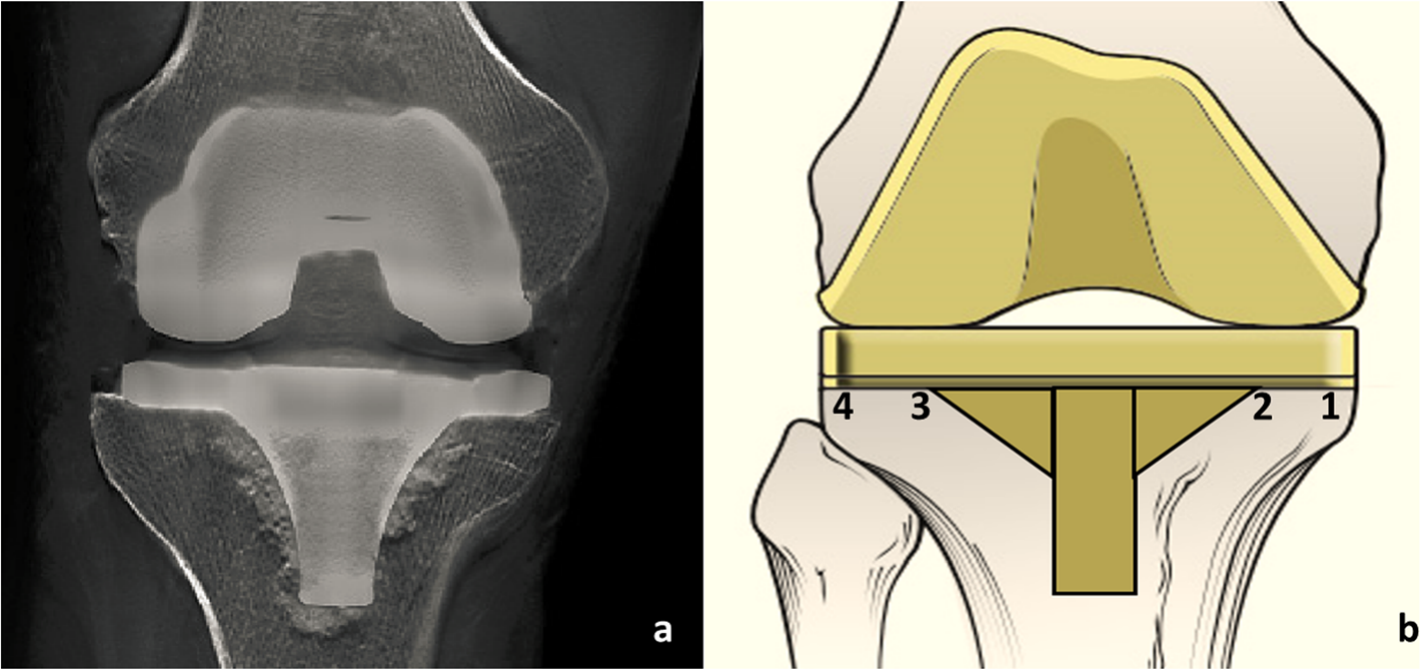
All patients received digital linear tomosynthesis (DTS) examiniation to measure bone cement mantle thickness. The measurement was performed based on the Knee Society scoring system. a. The radiographic film of digital linear tomosynthesis (DTS) examination, which showed better contrast than conventional DR film. b mantle thickness was measured in tibial baseplate based on the four zones
